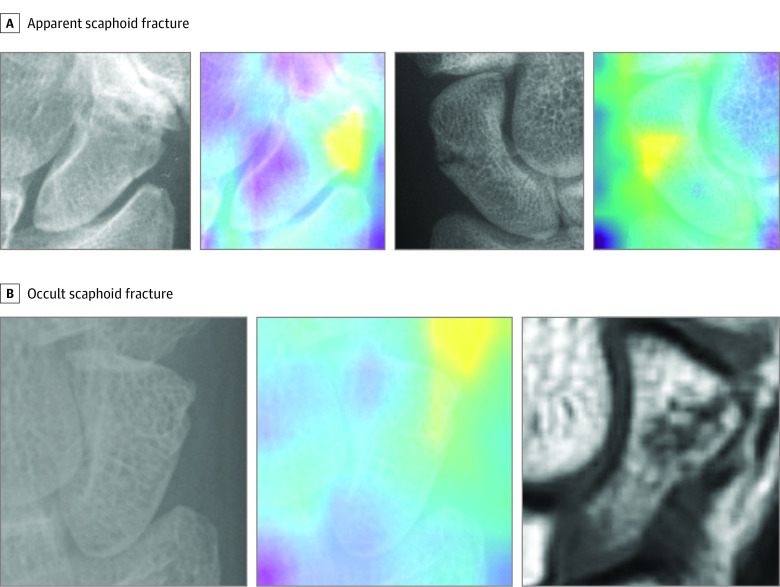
Localizing Fracture Sites Using Gradient-Weighted Class Activation Mapping (Grad-CAM) A, Example radiographs (first and third images) and Grad-CAMs (second and fourth images) for apparent scaphoid fractures. B, An example of the original radiograph (left), Grad-CAM (center), and confirmatory magnetic resonance imaging image (right) for occult scaphoid fractures. The model fracture predictions for apparent fractures (A) appear to be more precise than the predictions for occult fractures (B). For most occult fractures, the Grad-CAM images included the fracture line within the highlighted regions.
